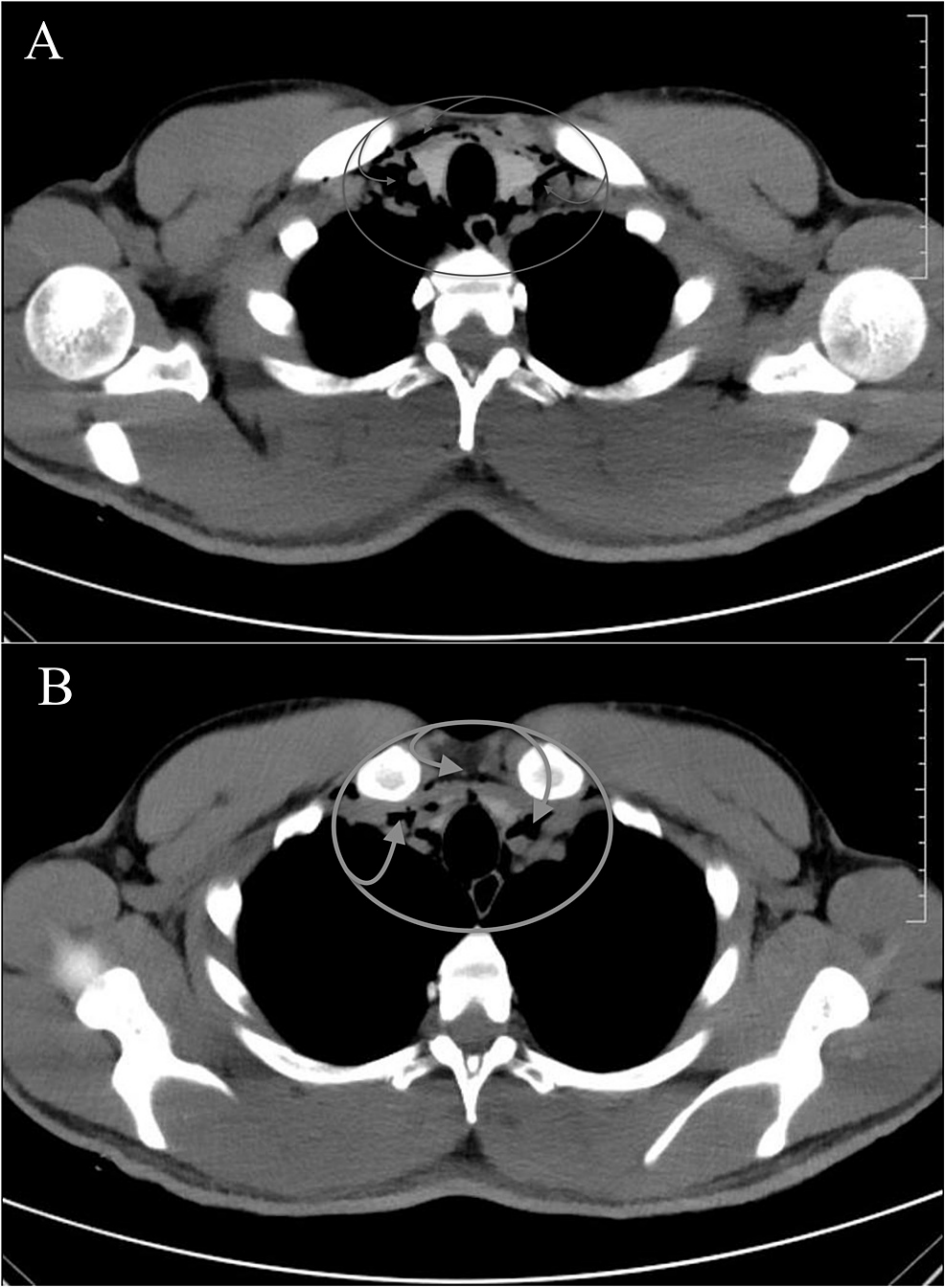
Multi-detector computed tomography scan of the patient’s chest demonstrating extensive pneumomediastinum and free air along the neck spaces, tracking to the retropharyngeal space and intermuscular plane of the neck, at slice 29 (A) and slice 32 (B).
